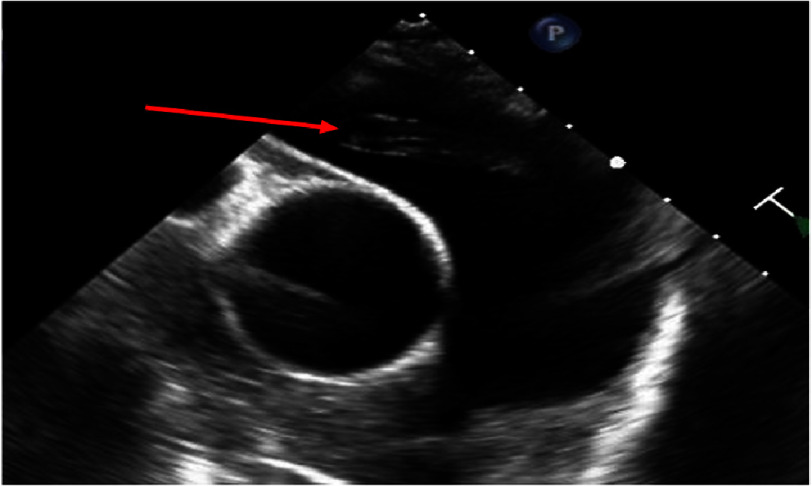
Transesophageal echocardiogram showing a long, mobile, serpiginous echodensity (arrow) from the pulmonary artery bifurcation to the right main pulmonary artery consistent with acute saddle PE.
